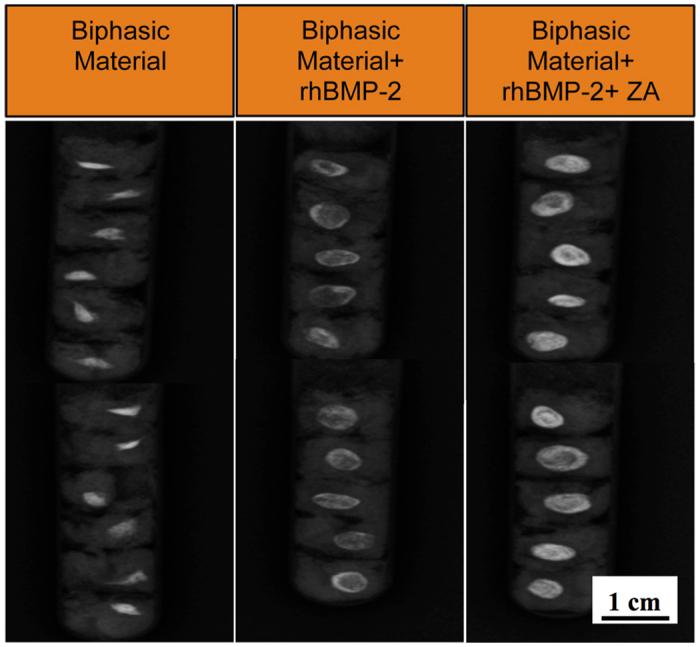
X-ray radiography of implanted samples in the abdominal muscle pouch. X-ray radiographs of biphasic material alone and combined with rhBMP-2 (10 μg) and rhBMP-2 (10 μg) + ZA (10 μg) in the abdominal muscle pouch after 28-days of *in-vivo* implantation. Scale bar represents 1 cm.
